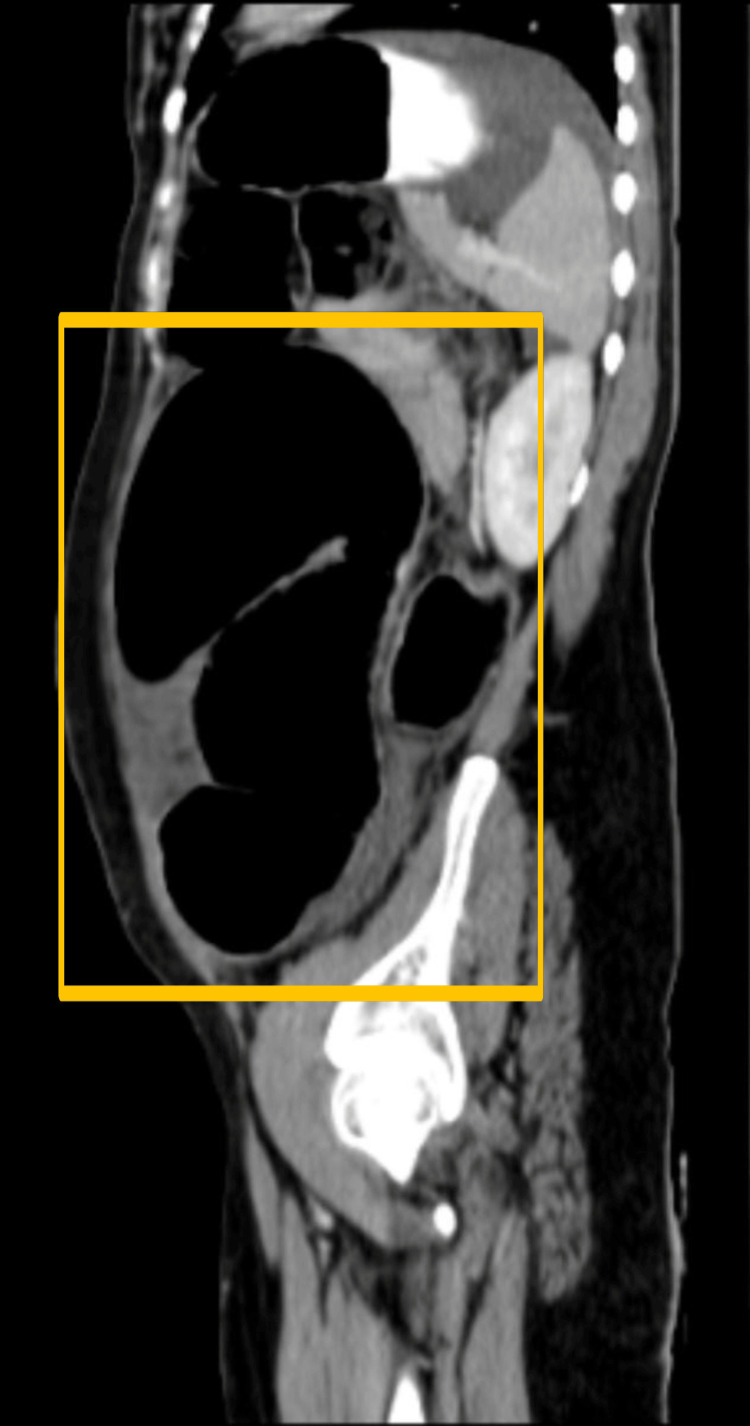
Sagittal section of the CT of the abdomen showing sigmoid volvulus
